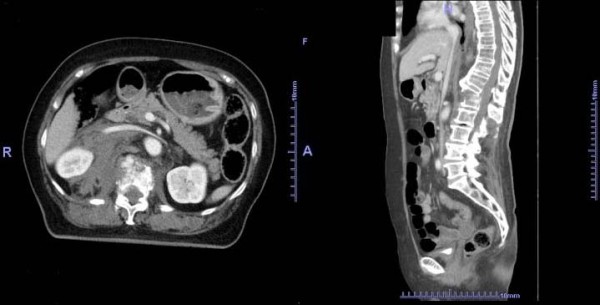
(a) Initial computed tomography (axial image:left); This CT scan shows a fracture of the first lumbar vertebra along with paravertebral and retroperitoneal hematomas. (b) Initial computed tomography (sagittal reconstruction:right); This CT scan shows a dislocation fracture (L1).
